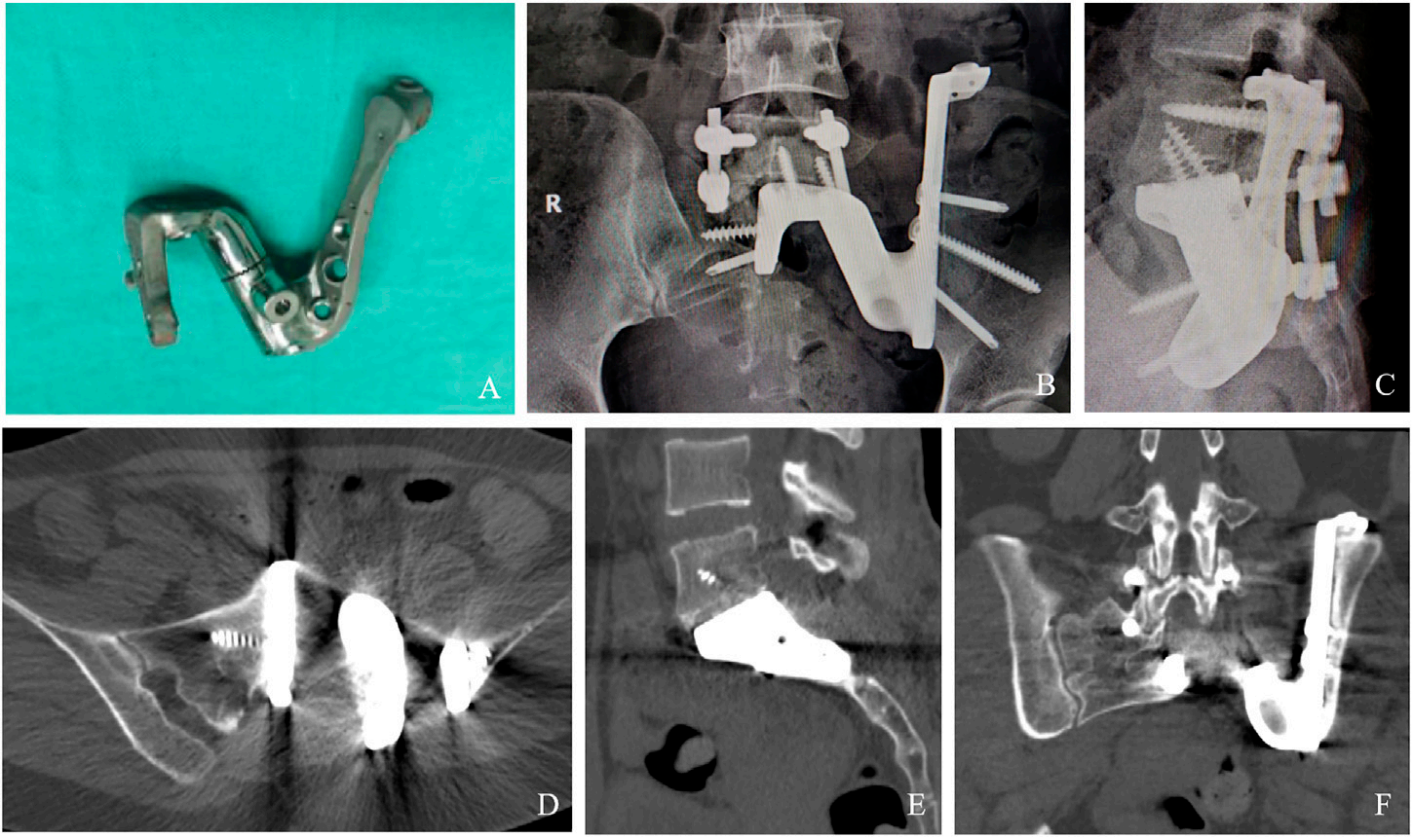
Results of prosthesis implantation. Physical object of the implant (A). X-ray examination 3 years after operation (B, C). CT showing excellent osseointegration at the bone–implant junctions in axial (D), sagittal (E), and coronal (F) views.
